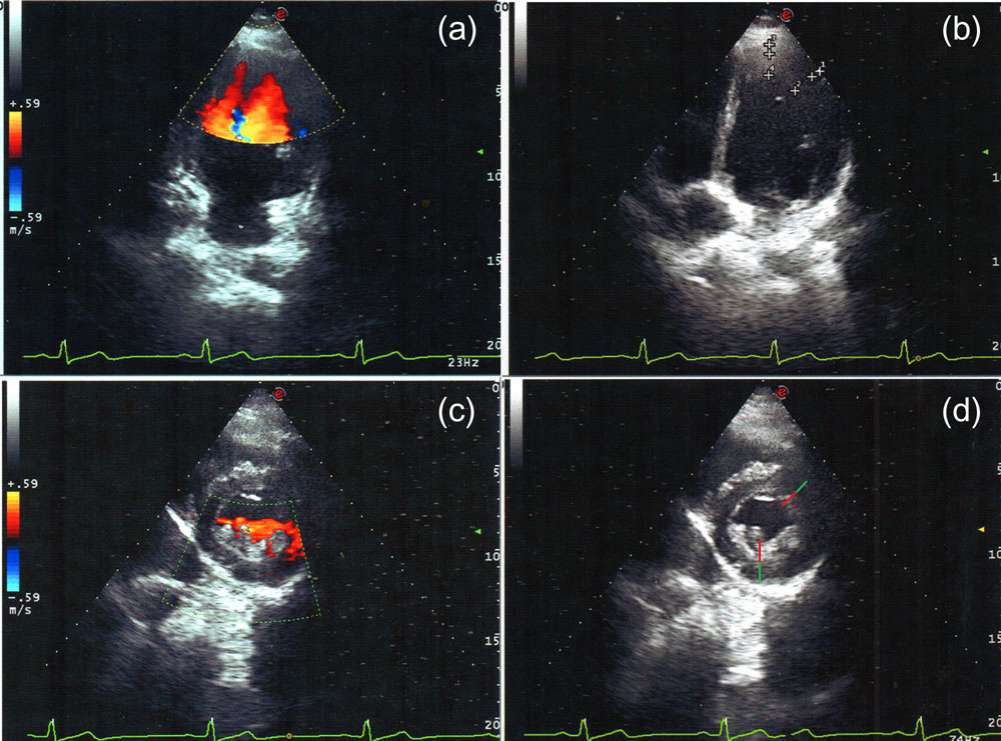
Echocardiogram: (a) Apical 4-chamber view, in color Doppler imaging where mild left ventricular dilatation is observed, noncompacted myocardium with trabeculations in apical wall; (b) Apical view where noncompacted myocardium (in red) and compacted myocardium ratio is observed (in green) ratio is >2; (c) Short axis left parasternal view; in color Doppler imaging multiple trabeculations and recesses observed in the lateral and inferior region, characteristic of noncompacted myocardium; (d) short axis left parasternal view the noncompacted myocardium (in red) and compacted myocardium (in green) ratio is >2.
